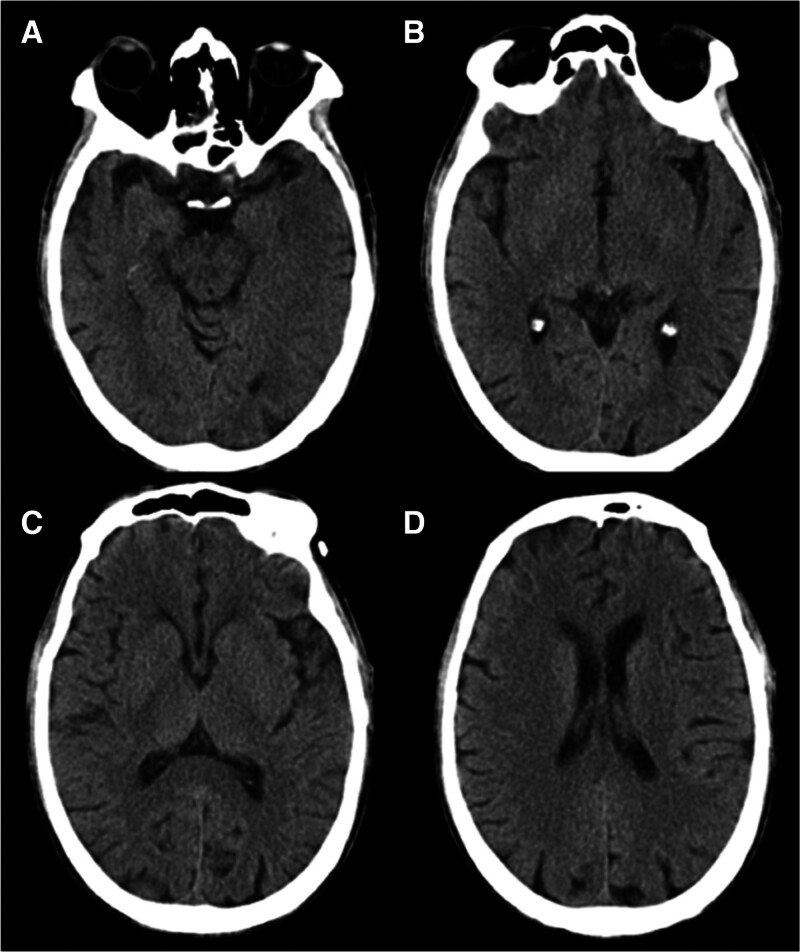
CT image of the patient. Cranial plain scan showed no abnormalities. (A) Midbrain level. (B) Third ventricle level. (C) Corpus callosum splenium level. (D) Lateral ventricle body level. CT = cranial computed tomography.
